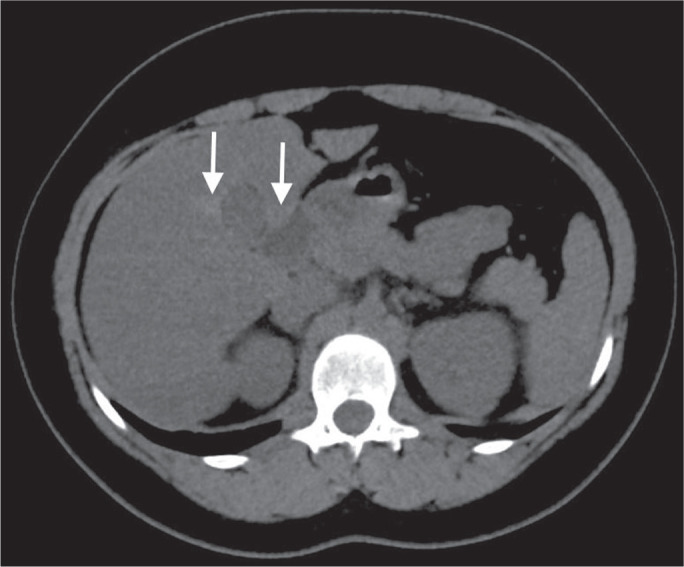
Unenhanced axial CT showing diffuse fat deposition with focal
